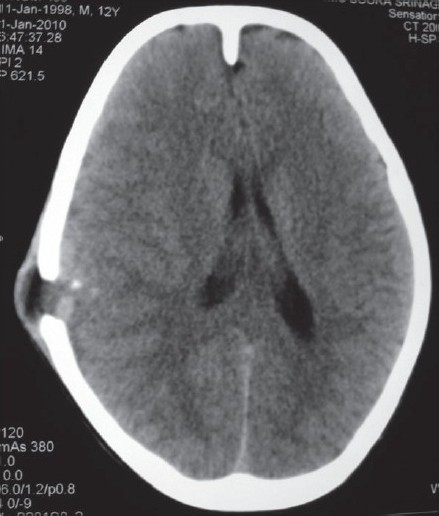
Plain CT-scan of head revealing erosion in the parietal bone with subperiosteal debris.
